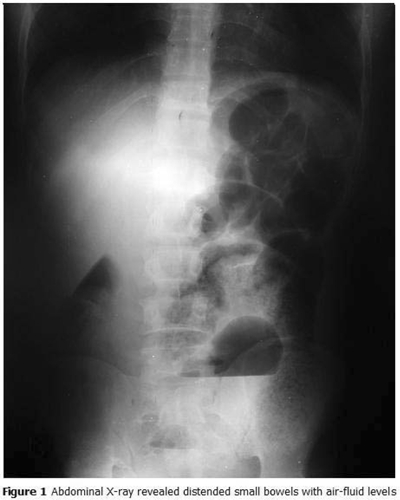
Abdominal X-ray revealed distended small bowels with air-fluid levels
